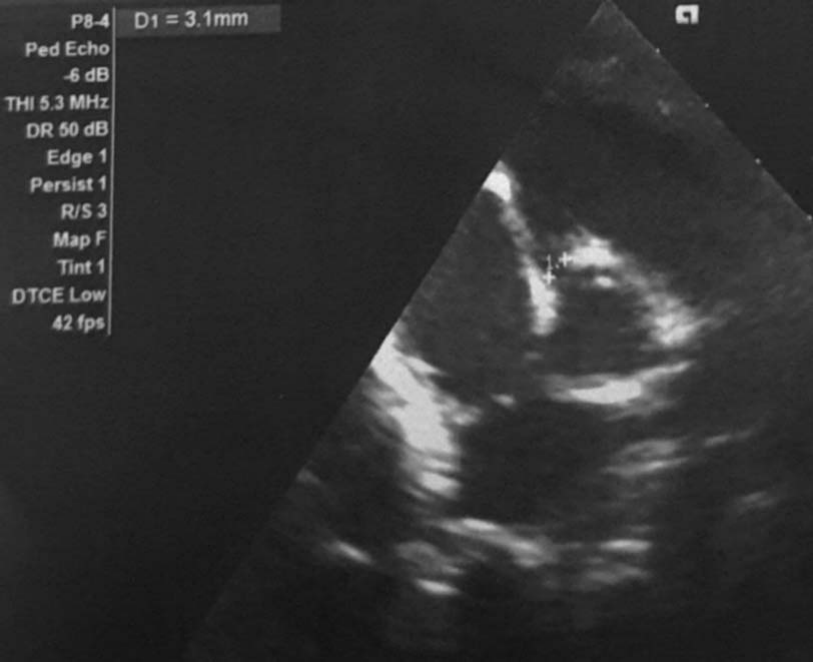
Transthoracic echocardiography showed dilation of the right coronary artery of 3.1 mm with a *z*-score of 4.05.
